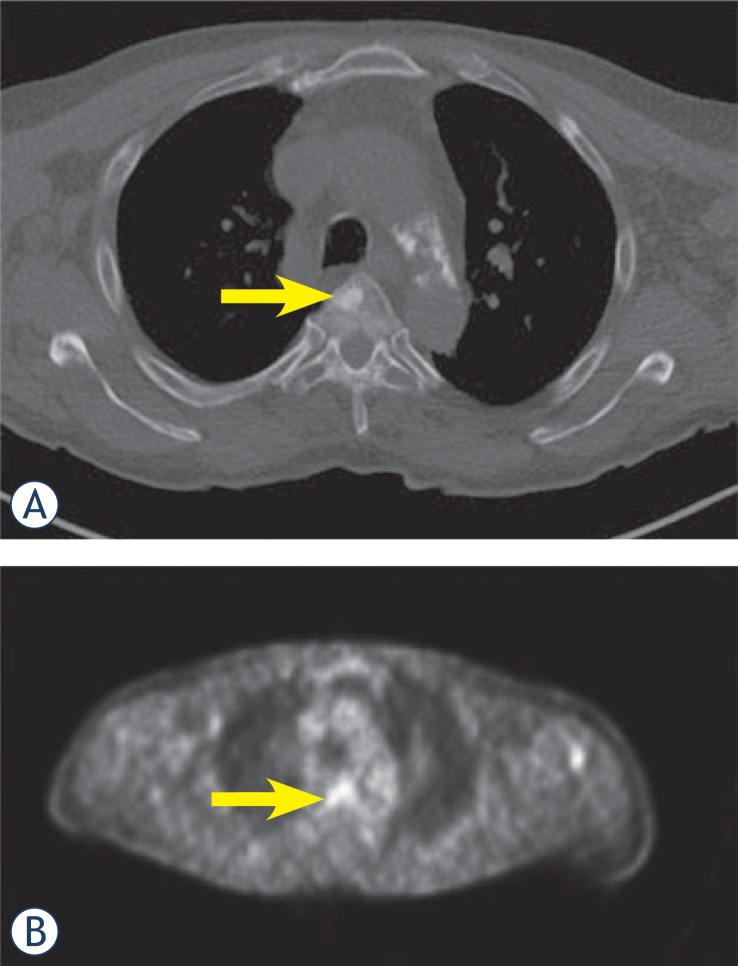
A. CT scan shows osteoblastic metastasis in the anterior part of thoracic vertebral body (arrow); B. PET-scan shows that the lesion is metabolically active (arrow).
